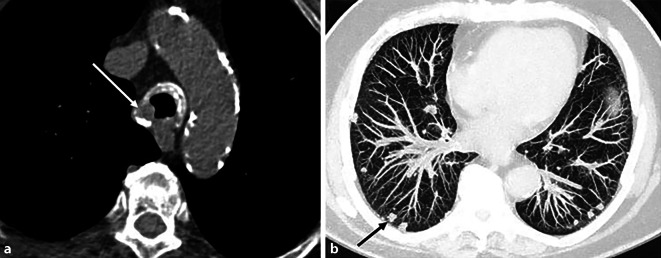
Tracheobronchiale und parenchymale Amyloidose-Manifestation. a CT-Thorax im Weichteilfenster eines 80-jährigen Patienten mit tracheobronchialer Manifestation einer pulmonalen Aspergillose. Bildmorphologisch zeigt sich eine zirkumferente tracheale Wandverdickung (Fortsetzung der Wandverdickung bis in die Hauptstammbronchien; hier jedoch nicht abgebildet) mit assoziierten, ausgedehnten submukosalen Kalzifikationen. Zudem plaqueartige, submukosale Trachealauflagerung rechts-laterodorsal (*weißer*
